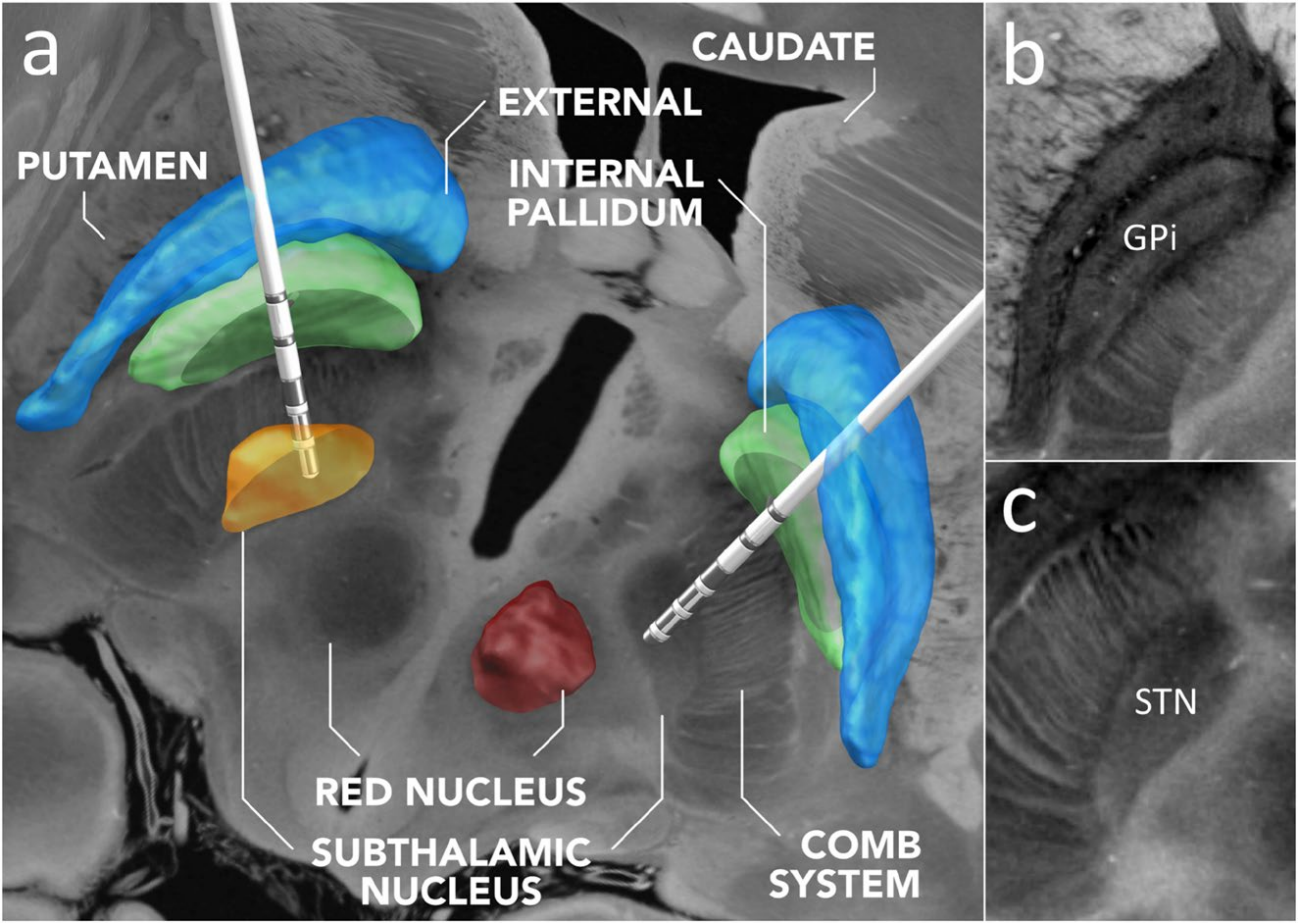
Normalization of the *ex vivo* MRI dataset into standard stereotactic space and integration into the Lead-DBS software platform. (a) Exemplary use-case of the normalized FLASH25 volume in deep brain stimulation (DBS). DBS electrodes are visualized for a hypothetical patient using Lead-DBS software (https://www.lead-dbs.org)^31^. An axial image from the normalized scan, at the level of the rostral midbrain, is shown as a backdrop, with 3D-structures defined by the DISTAL atlas^35^ (right subthalamic and left red nucleus hidden for optimal visualization of the underlying anatomy). Panels (b) and (c) show zoomed views of key DBS target regions: the left globus pallidus interna (GPi in b) and subthalamic nucleus (STN in c).
